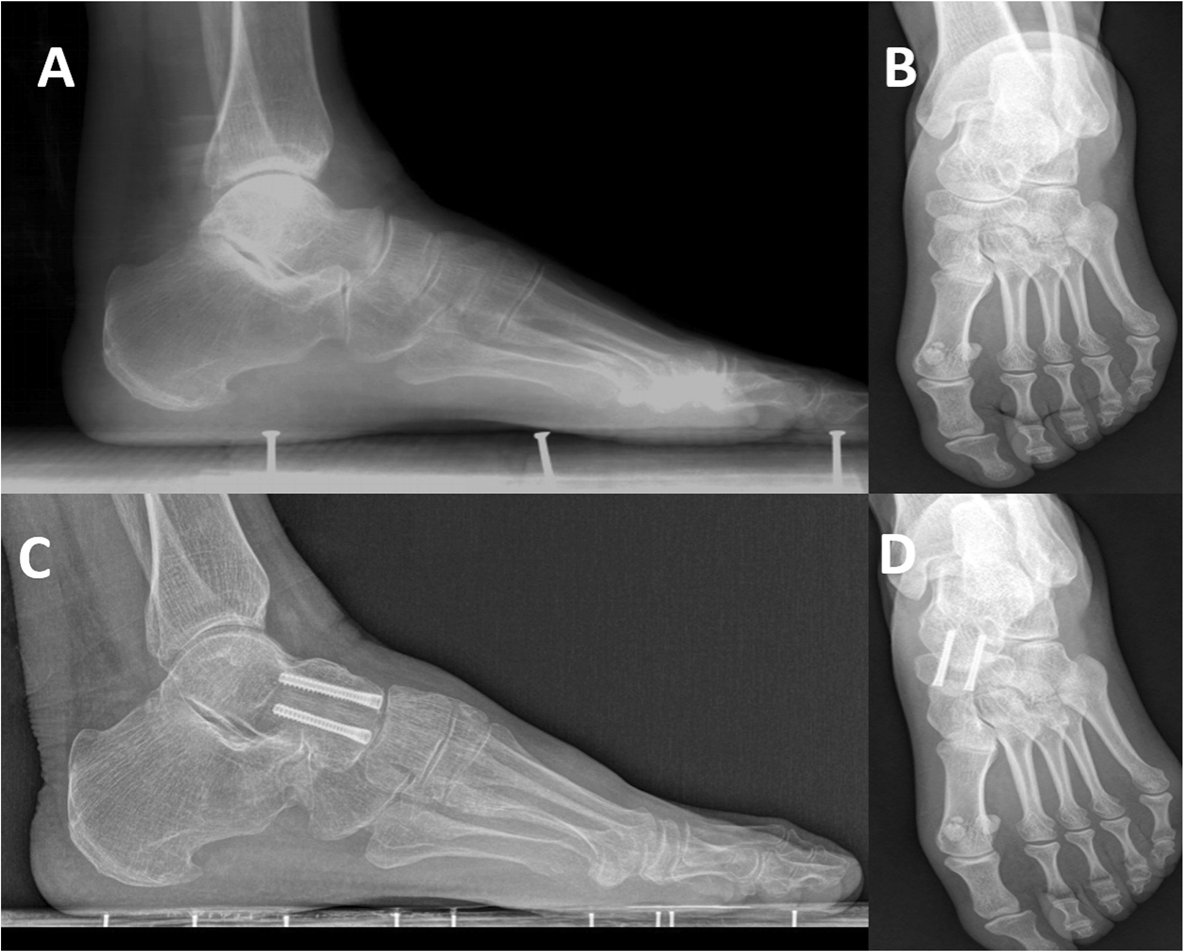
Preoperative (a, b) and postoperative (c, d) radiographs to TNA with double HCSs after 4 years
